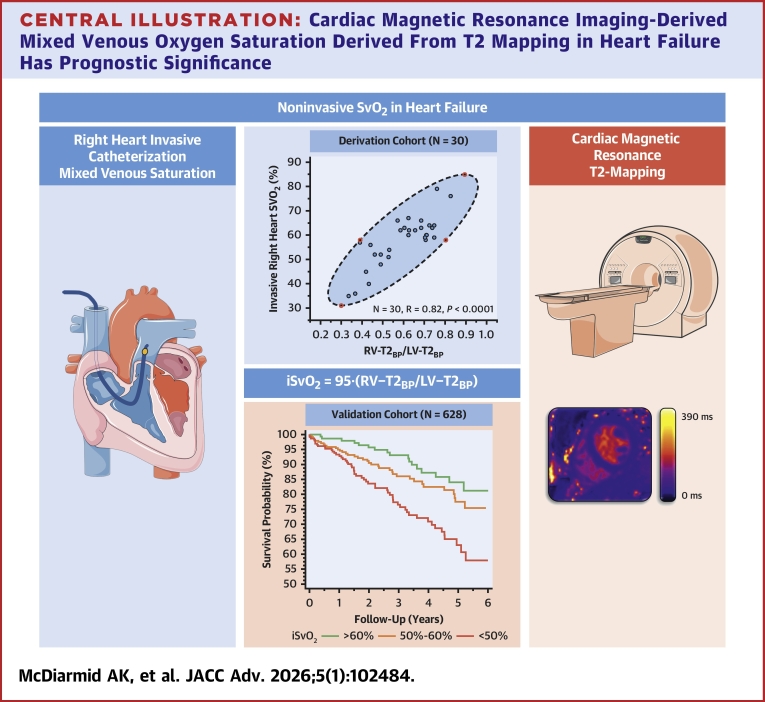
Cardiac Magnetic Resonance Imaging-Derived Mixed Venous Oxygen Saturation Derived From T2 Mapping in Heart Failure Has Prognostic Significance iSvO_2_ = imaging-derived mixed venous oxygen saturation; LV-T2_BP_ = left ventricular blood pool T2 value; RV-T2_BP_ = right ventricular blood pool T2 value; SvO_2_ = mixed venous oxygen saturation.
